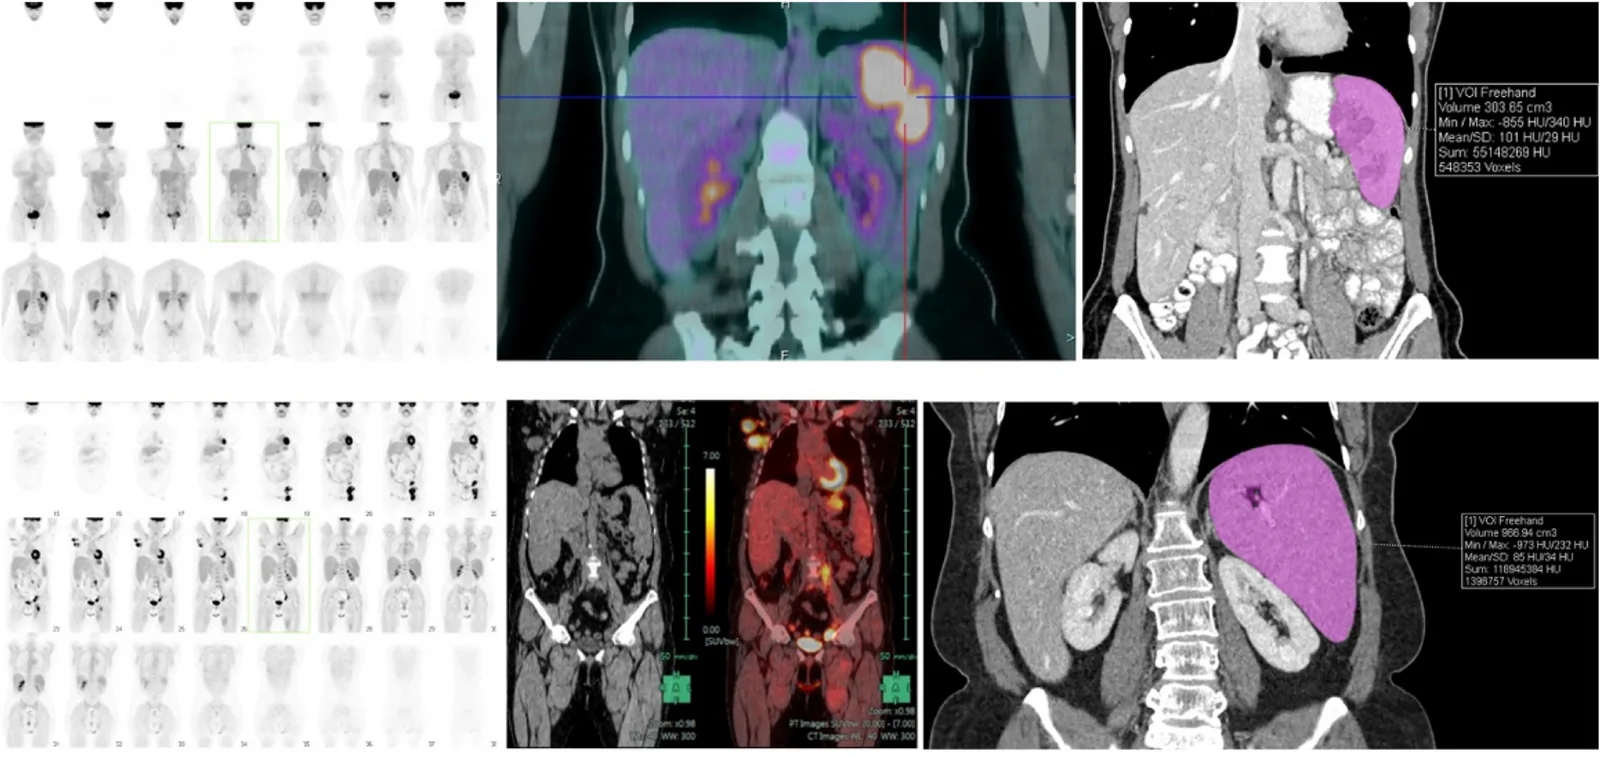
Baseline PET/CT and contrast-enhanced CT (CECT) in two patients with DLBCL. (a–c) PET-positive spleen without splenomegaly: (a) whole-body PET maximum intensity projection, (b) coronal PET/CT fused image, (c) coronal CECT with spleen segmentation showing normal volume (3.03 dL). (d–f) PET-negative spleen with marked splenomegaly: (d) whole-body PET maximum intensity projection, (e) coronal PET/CT fused image, (f) coronal CECT with spleen segmentation showing splenic enlargement (9.67 dL). PET = positron emission tomography; CT = computed tomography; CECT = contrast-enhanced CT; DLBCL = diffuse large B cell lymphoma
